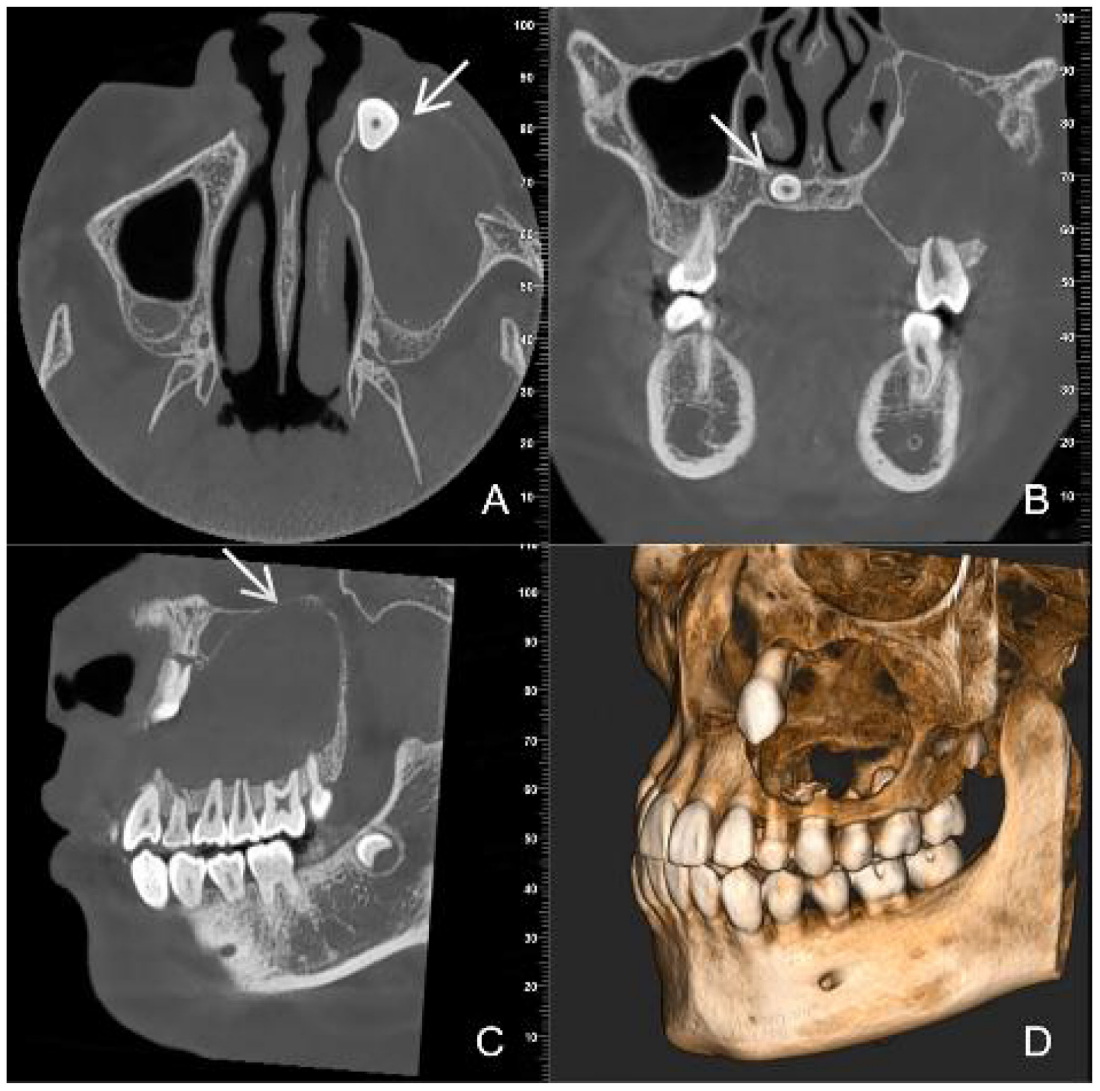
Preoperative CBCT images (A) (axial view) (B) (coronal view) (C) (sagittal view) (D) (three-dimensional reconstruction) show a large, low- density cyst with clear boundaries [images (C) white arrow]. An impacted tooth 23 [images (A) white arrow] with large irregular high-density masses is present within the lesion. A supernumerary tooth [images (B) white arrow] is observed in the maxillary palate slightly to the right of the midline.
